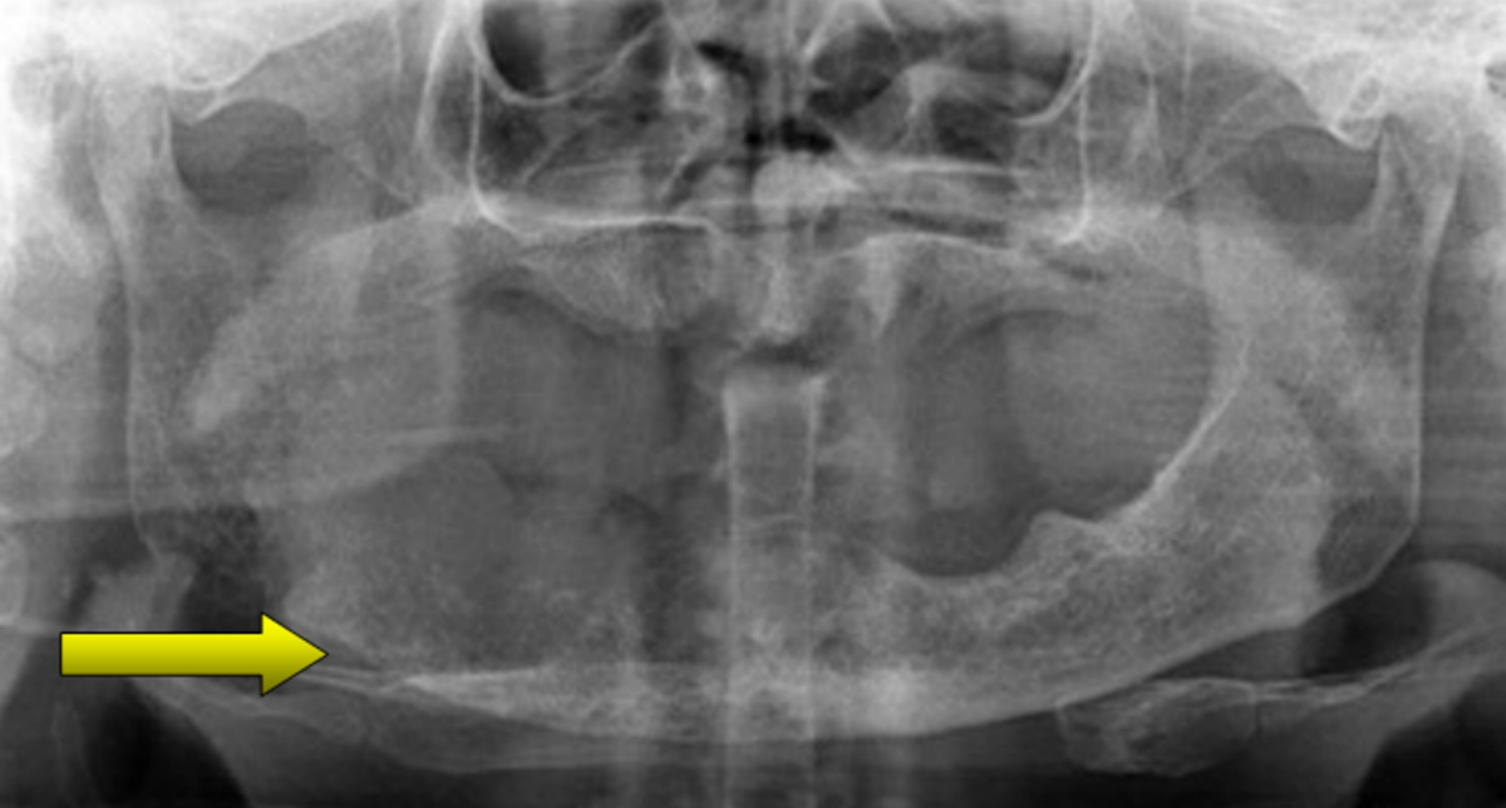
Preoperative orthopantomogram showing radiolucent areas in the right body and ramus of the mandible indicating erosions
